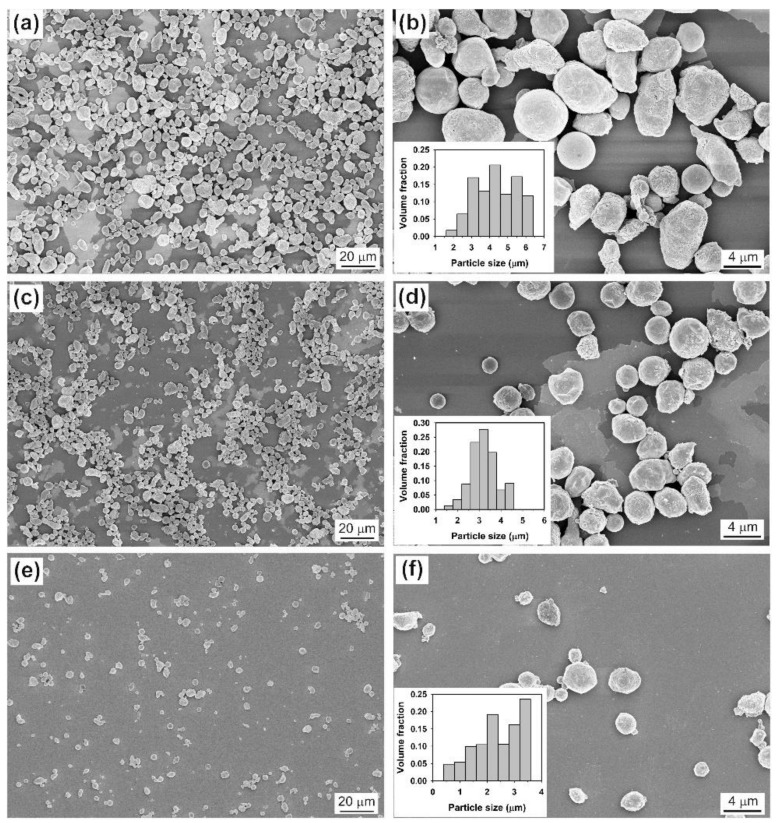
SEM images of the products obtained in LiNO_3_-NaNO_3_-KNO_3_ system under different sonication times at an ultrasonic power of 12 W: (a,b) 1 min, (c,d) 2 min, and (e,f) 5 min. Other conditions: volume ratio of salts to metal Sn = 1000:1. Inset is the corresponding volume-based particle size distribution.
